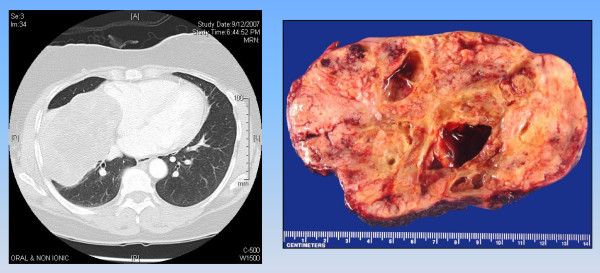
Radiographic and gross pathologic appearance of primary pulmonary myoepithelial carcinoma. A. Computed tomography of right-sided chest mass with compression of right atrium. B. Gross pathologic appearance of right visceral pleural mass.
